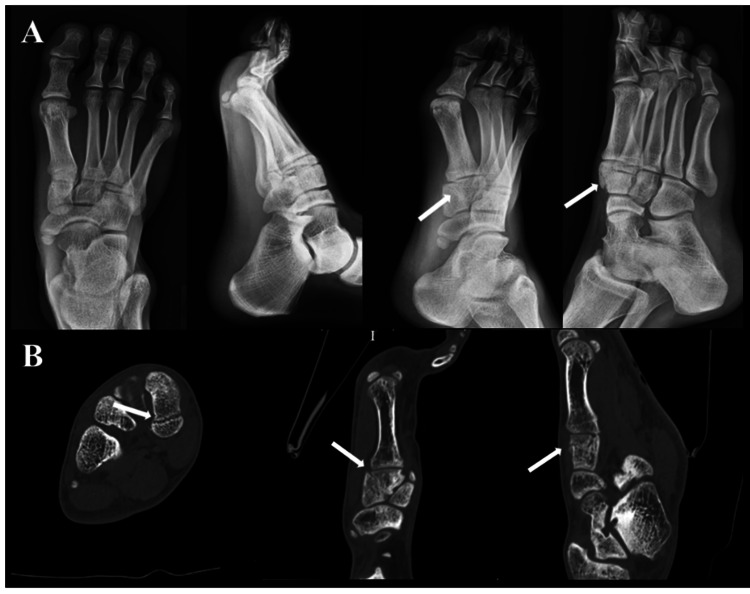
Preoperative images. A: Radiograph; B: CT (White arrows indicate the fracture line)
